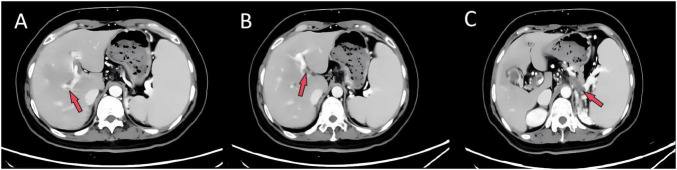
Contrast-enhanced abdominal CT. (A) Right portal vein branch, (B) left portal vein branch, and (C) splenic vein all reveal filling defects, suggesting widespread thrombosis.
